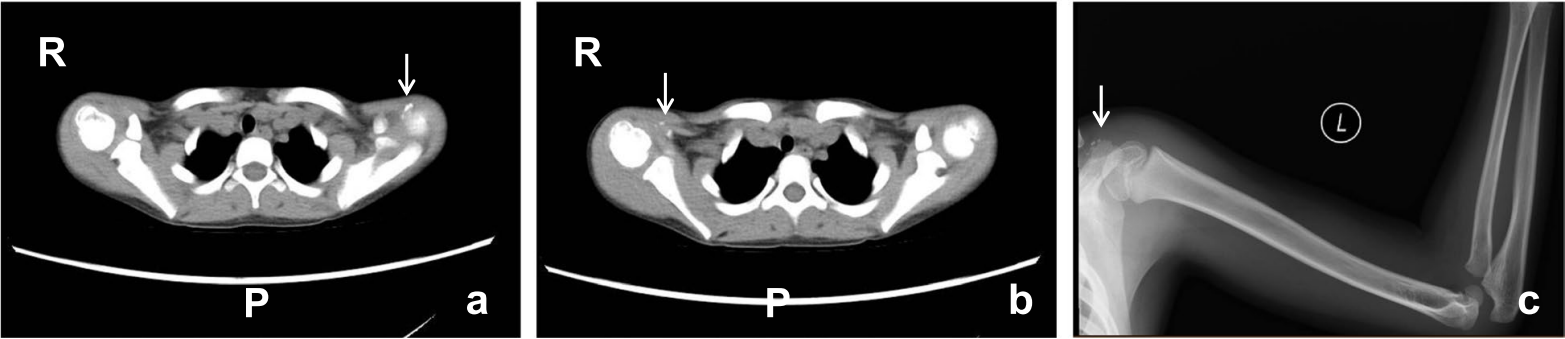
The radiographic image of the shoulders. The radiographic image of the shoulder revealed tiny radiodensities (arrows) seen in the skeletal muscle of bilateral proximal humerus (a, b) and left acromial area (c)
